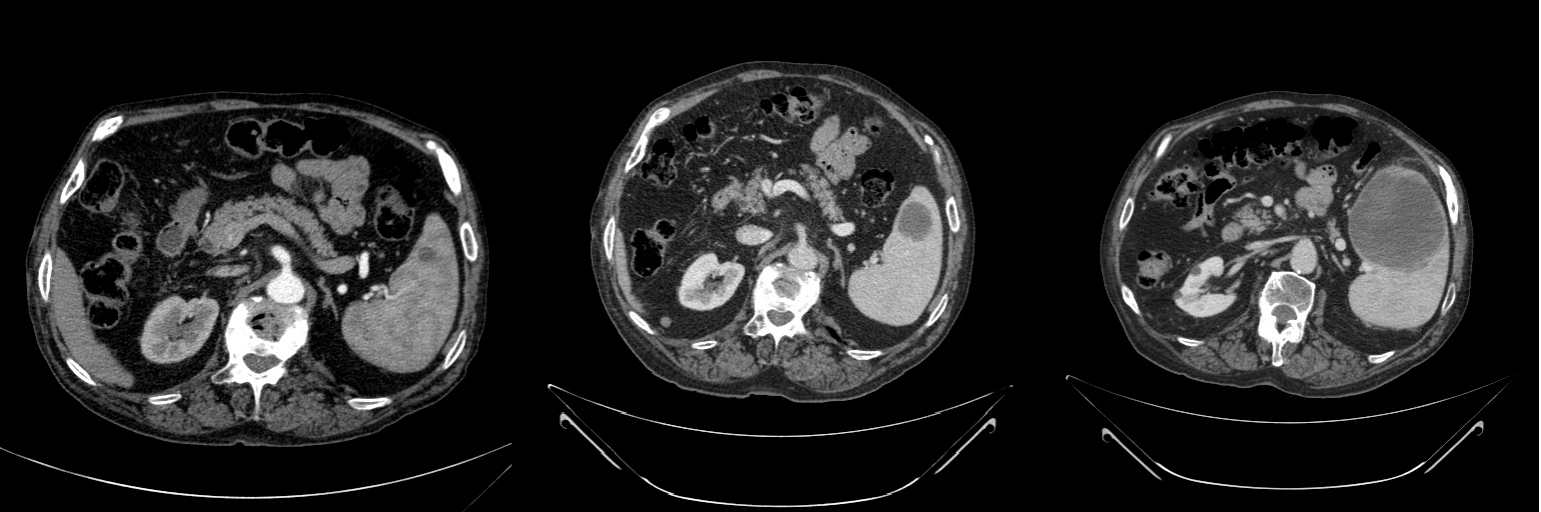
CT scan of the splenic metastasis at postoperative months 16, 28 and 30 (right to left).
